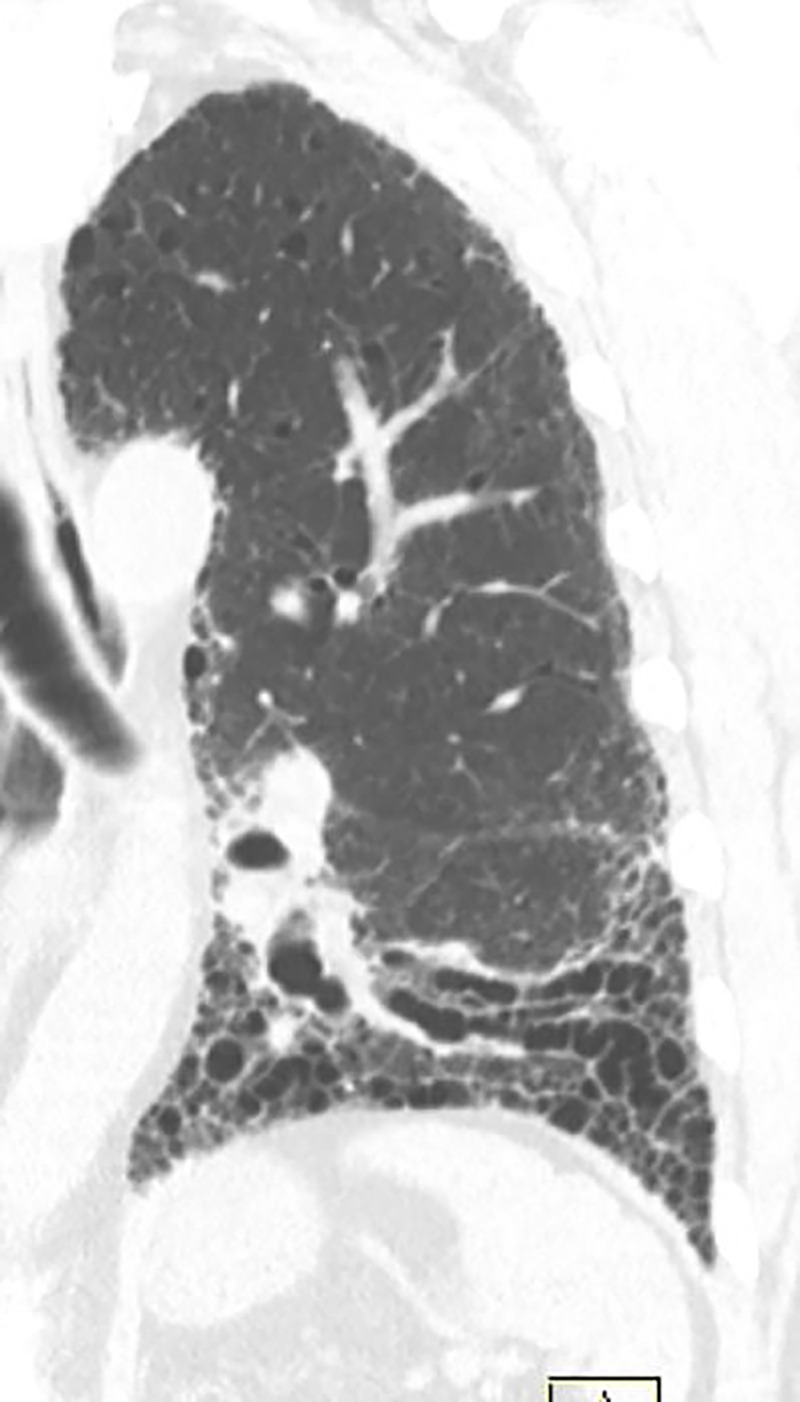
Traction bronchiectasis and bronchiolectasis. Zoomed view of coronal CT demonstrates continuity of dilated irregular airways with the more central bronchi (traction bronchiectasis and bronchiolectasis) in a patient with fibrotic nonspecific interstitial pneumonia.
